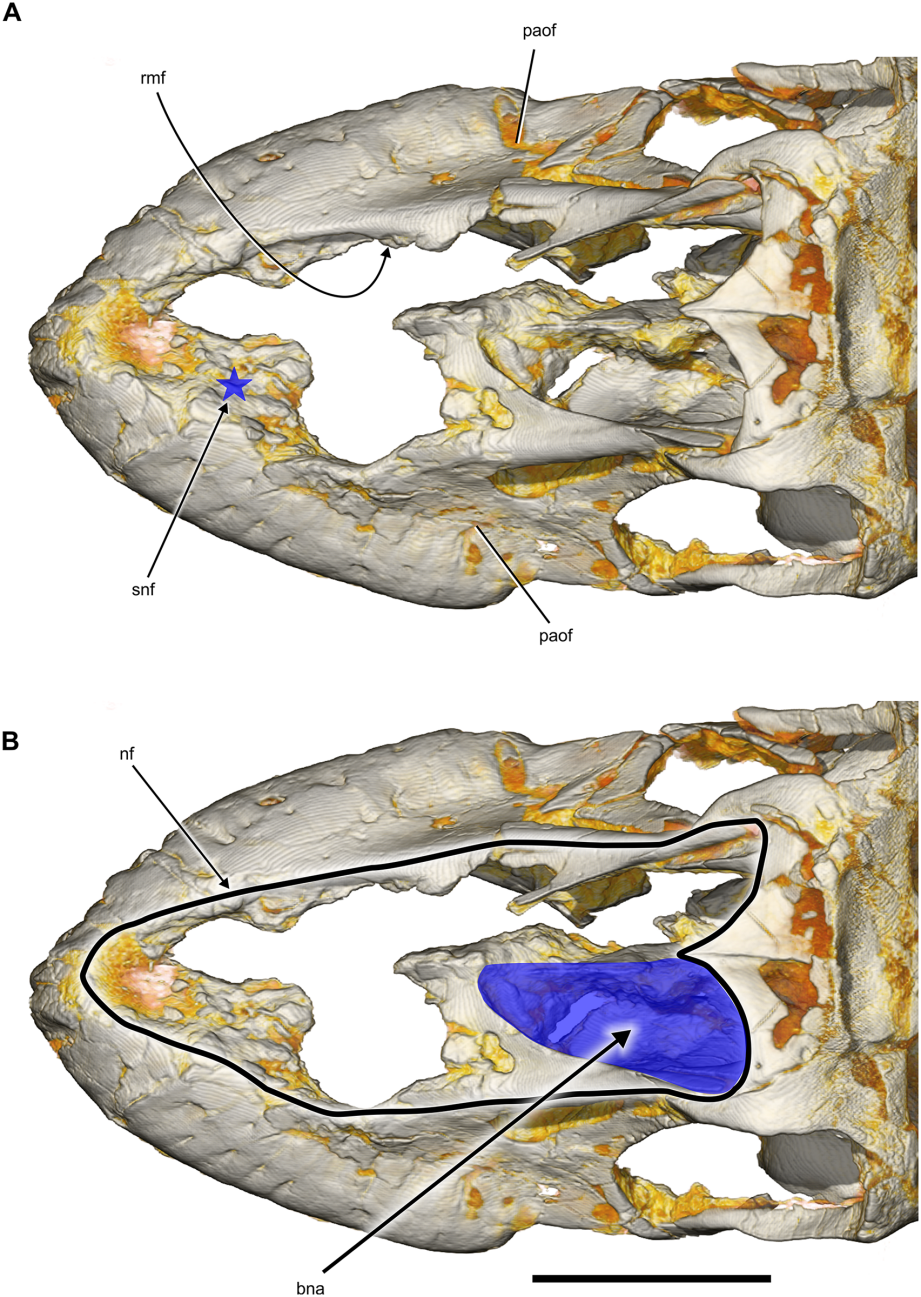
Snout and narial region of *Sarmientosaurus musacchioi* gen. et sp. nov. (MDT-PV 2). Computed tomography-based digital visualization in dorsal view, showing locations of preantorbital, rostral maxillary, and subnarial (blue star) foramina (A), and left bony nasal aperture (blue overlay) and narial fossa (black line) (B). Abbreviations see text. Scale bar = 10 cm.
